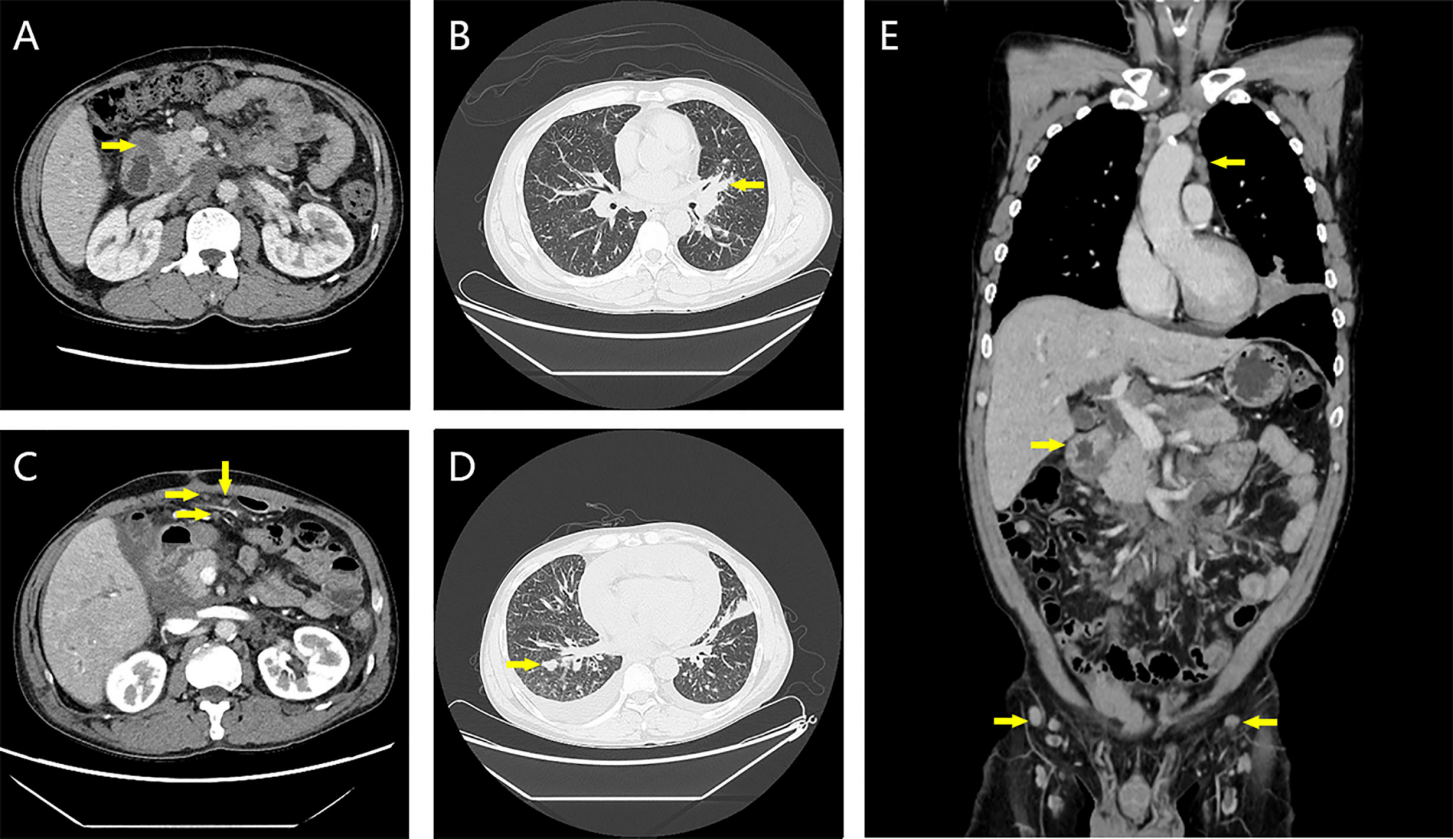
Contrast-enhanced CT images at first admission and 2 weeks after three cycles of chemotherapy. (A) The wall of the descending duodenum was thickened at the first admission. (B) Bronchus occlusion in the lingual segment of the left upper lobe of the lung on the first admission. (C) Irregular thickening of partial peritoneum, omentum, and mesentery at 2 weeks after the third cycle of chemotherapy. (D) New solid nodule in the right lung 2 weeks after the third cycle of chemotherapy. (E) On the first admission, thickening of the intestinal wall of the descending duodenum and multiple mediastinal and inguinal lymphadenopathy were seen.
